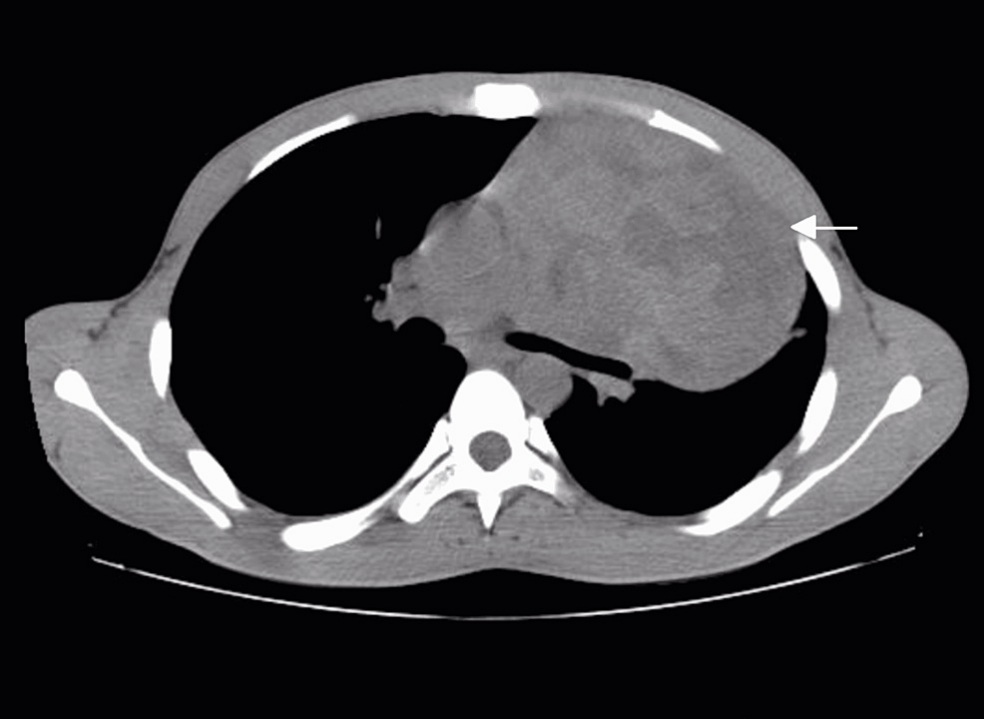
Chest computed tomography showing a well-defined, heterodense anterior mediastinal mass occupying the left hemithorax
